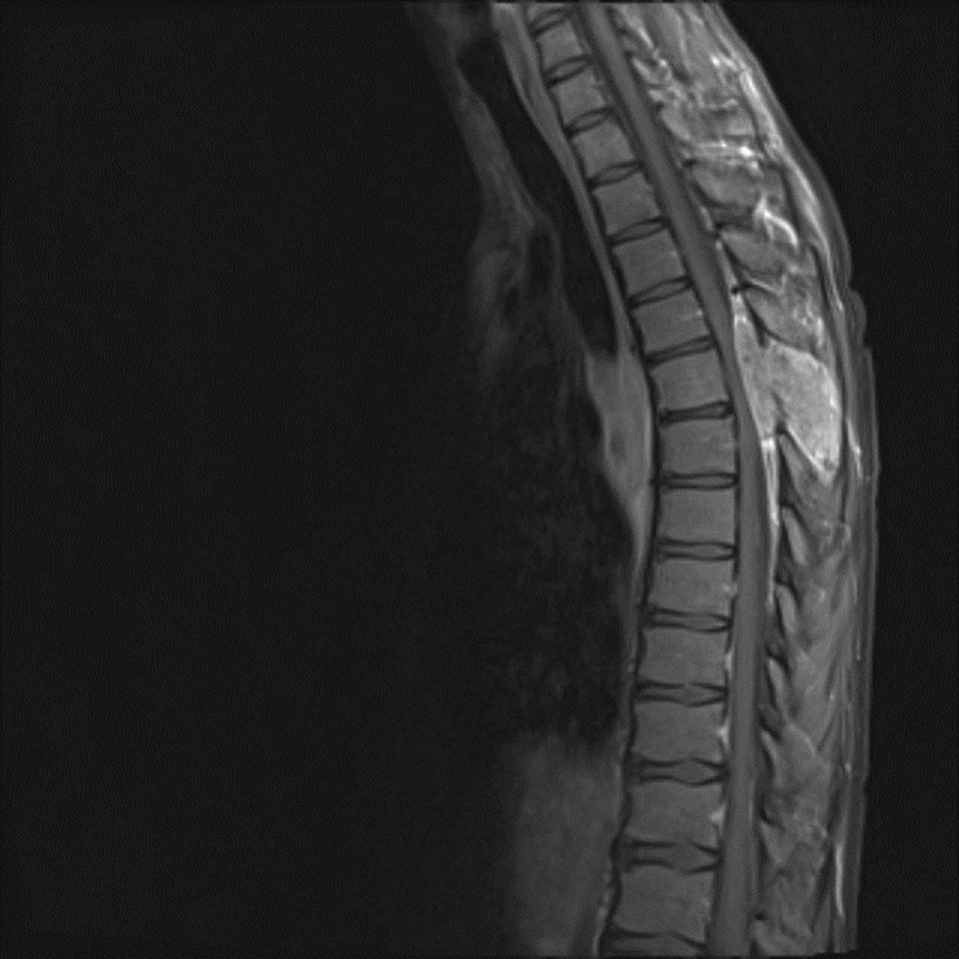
MRI of the thoracic spine, in sagittal view, T1-weighed image with contrast medium enhancement. The tumor leads to significant myelocompression, and grows infiltratingly into the surrounding tissue.
